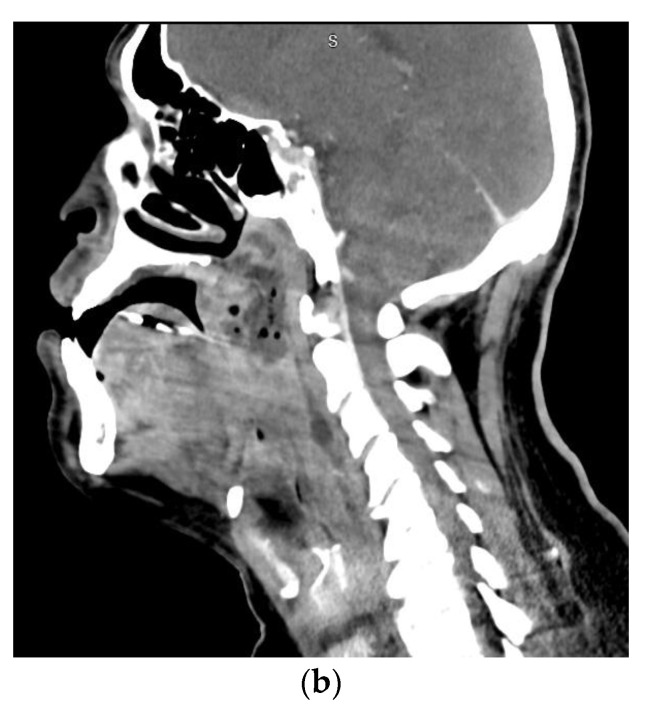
Axial (a) and sagittal (b) computed tomography scan views of a patient with a left parapharyngeal space infection with significant gas formation. Air and fluid extended within the retropharyngeal space to the level of C6/7. Bacteremia was present, with blood cultures positive for Streptococcus anginosus.
